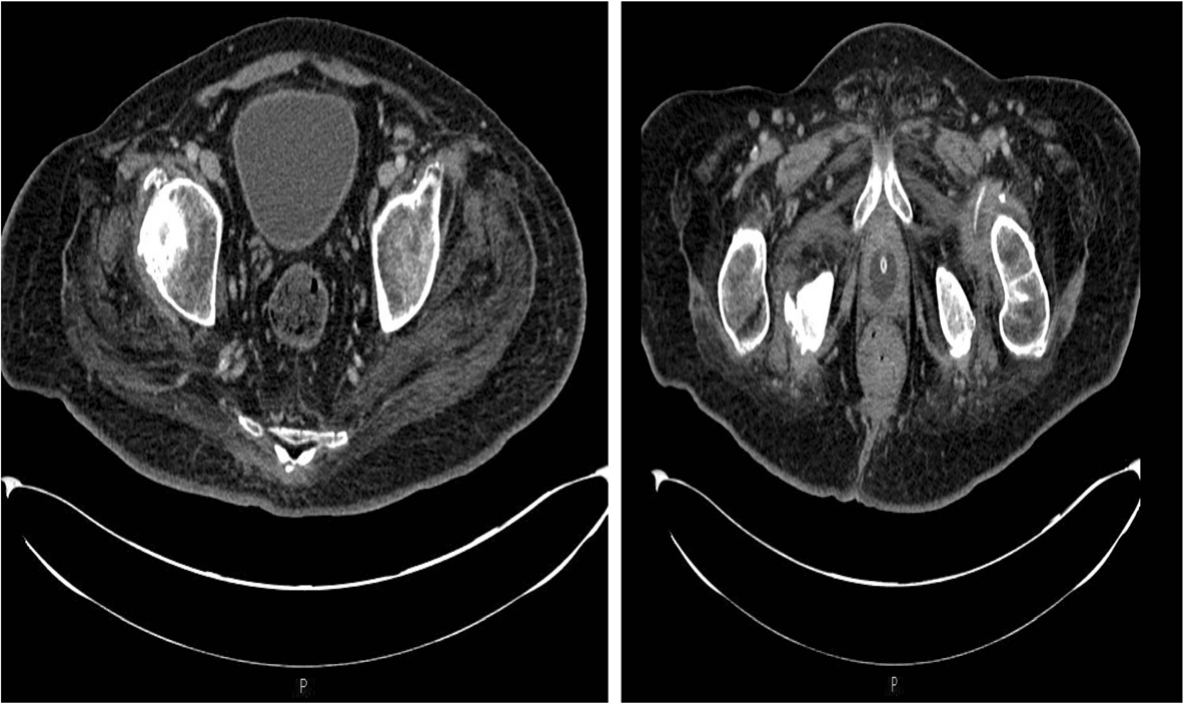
Case 4. Left panel: Axial section of CT of abdomen and pelvis revealed full bladder; no catheter was seen in the lumen of urinary bladder. Right panel: Axial section of CT of pelvis revealed balloon of Foley catheter in the proximal urethra within prostate
